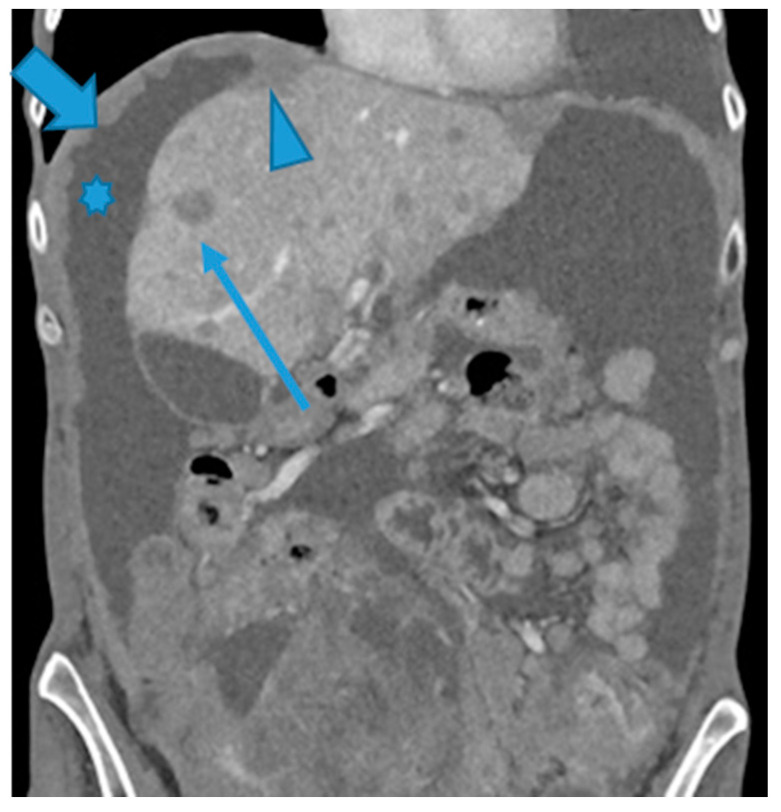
CE-CT coronal MPR. PC from ovarian carcinoma: multiple bilateral diaphragmatic nodular deposits (arrows). Notice how useful ascites (*) is when distinguishing peritoneal deposits within the parietal peritoneum from deposits within the visceral (hepatic) peritoneum (arrowhead). Hepatic metastases (thin arrow).
